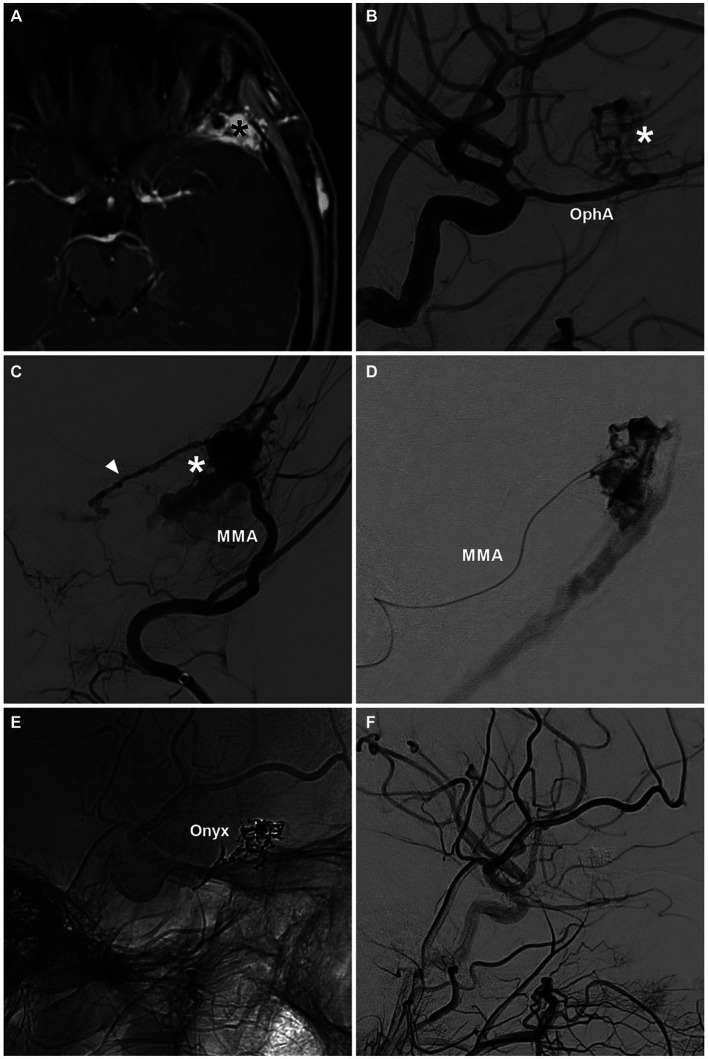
Complete EVT via the MMA for a sphenoidal region DAVF. (A) MRI image showing a high signal (asterisk) at the left sphenoidal ridge. (B) DSA image showing the DAVF (asterisk) supplied by the recurrent meningeal branch of the OphA. (C) DSA showing a sphenoidal ridge DAVF (asterisk) supplied by the MMA. The arrowhead indicates the anastomosis with the recurrent meningeal branch of the OphA. (D) Selective angiography of the MMA showing the DAVF. (E) Unsubtracted DSA showing the Onyx casting. (F) Post-EVT DSA showing that the DAVF was obliterated. DAVF, dural arteriovenous fistula; DSA, digital subtraction angiography; EVT, endovascular treatment; MMA, middle meningeal artery; MRI, magnetic resonance imaging; OphA, ophthalmic artery.
